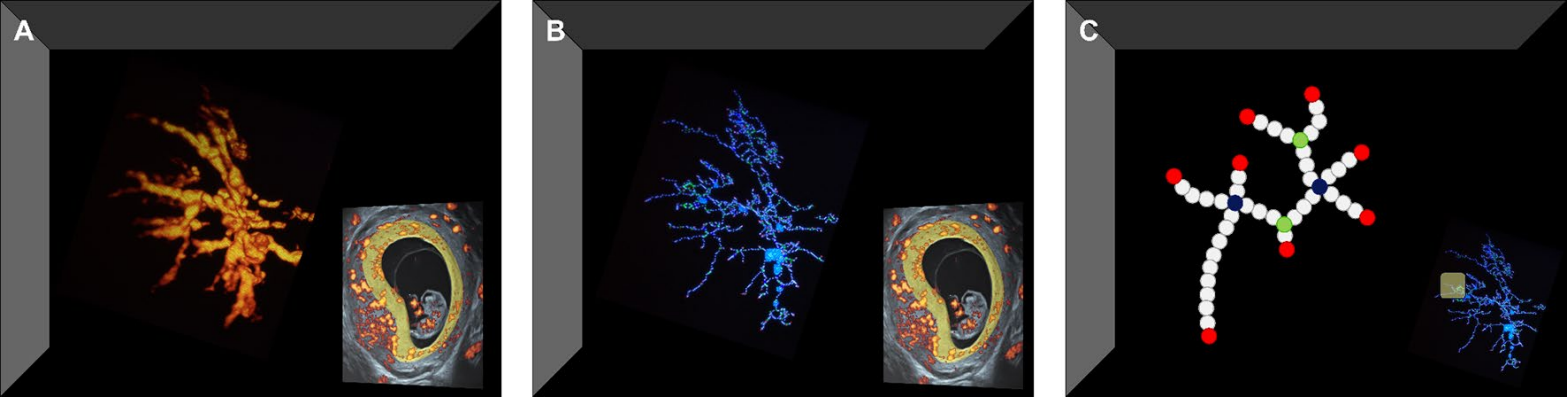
Overview of utero-placental (vascular) imaging markers; the placental volume (PV), utero-placental vascular volume (uPVV) and utero-placental vascular skeleton (uPVS) of a first-trimester pregnancy. A: Utero-placental vascular volume (uPVV) and slice view of a three-dimensional (3D) power Doppler (PD) ultrasound of a first trimester pregnancy (bottom right). The yellow-highlighted section in the bottom right image indicates placental tissue, which is confined by the placental-myometrial interface on the outside and the gestational sac on the inside. These anatomical boundaries are used to calculate the placental volume (PV). Using virtual reality-based segmentation, all PD signals outside the PV-segment were erased. The uPVV is calculated by summation of the thresholded 3D PD voxels (3D pixels) within the PV-segment. B: Utero-placental vascular skeleton (uPVS) and slice view of a three-dimensional (3D) power Doppler (PD) ultrasound of a first trimester pregnancy (bottom right). A skeletonization algorithm has been applied to the uPVV (from panel A), resulting in the uPVS. The skeletonization algorithm repeatedly peels off the outermost layer of voxels from the uPVV, reducing the diameter of the PD signal at each point in the vascular network until one central voxel remains, thereby creating a network-like structure representing the vascular morphology. C: A magnified portion of the uPVS and overview of the uPVS including the magnified portion (yellow-highlighted section) at the bottom right. Each voxel of the uPVS is automatically assigned a morphologic characteristic based on the number of adjacent voxels: red = endpoint (1 adjacent voxel); white = vessel point (2 adjacent voxels); green = bifurcation point (3 adjacent voxels); blue = crossing point (4 adjacent voxels). Two other characteristics are derived from the uPVS: 1. Total network length was calculated by summation of the total number of voxels in the skeleton, multiplied by 1 voxel length (mm). 2. Average vessel thickness was calculated by the average number of voxel-layers that were peeled off from the uPVV to reach the central voxel of the uPVS, multiplied by 1 voxel length (mm)
